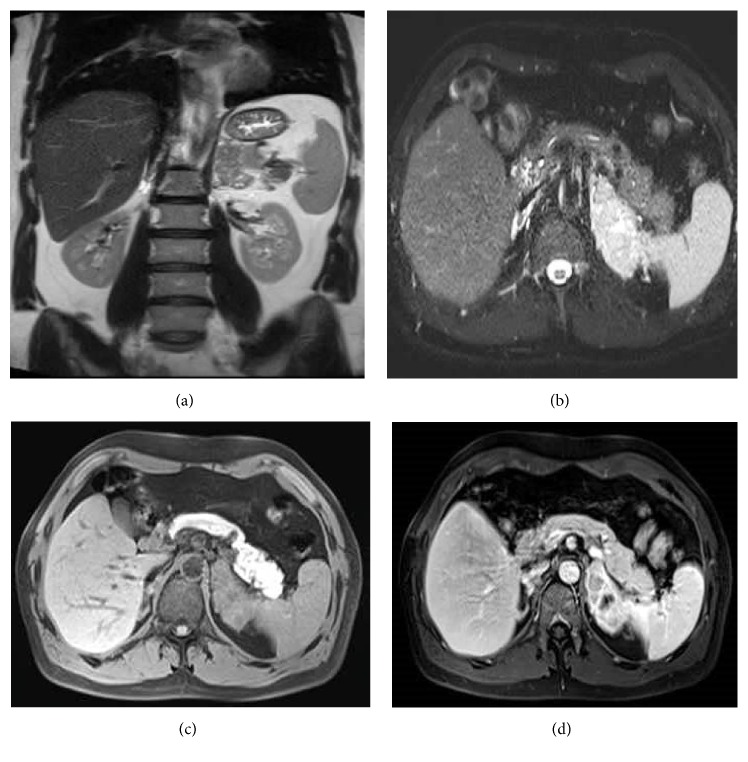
(a) Coronal T2-weighted and (b) axial fat saturated T2-weighted images show slightly hyperintense left adrenal gland lesion by comparison to spleen, with cystic degenerative components. (c) Axial fat saturated nonenhanced T1-weighted and (d) axial fat saturated enhanced T1-weighted enhanced images reveal diffuse and heterogeneous enhancement of lesion.
